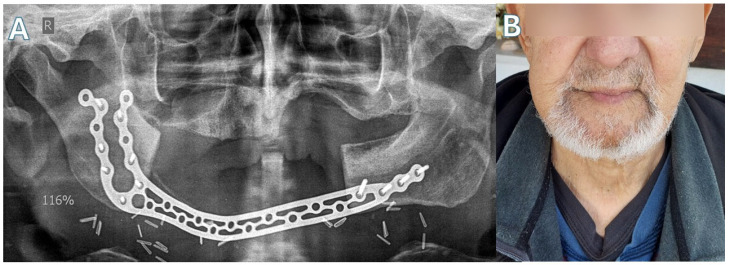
Case 3. (A) Post-op panoramic X-ray. (B) The patient 20 months after surgery.
